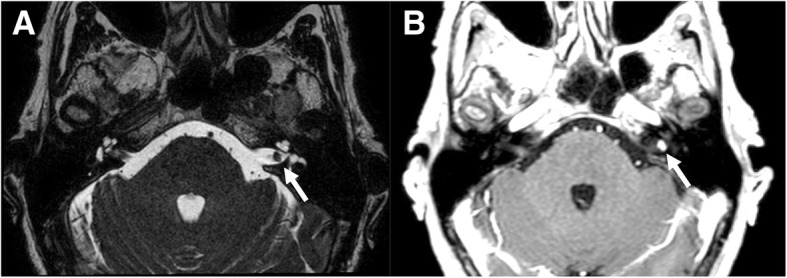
Small intracanalicular vestibulocochlear schwannoma. MRI steady-state free procession (SSFP) (a) and T1-weighted post-gadolinium (b) axial images show a small hypointense lesion on steady-state sequence with avid contrast-enhancement visible in the intracanalicular segment of internal auditory canal (arrows)
